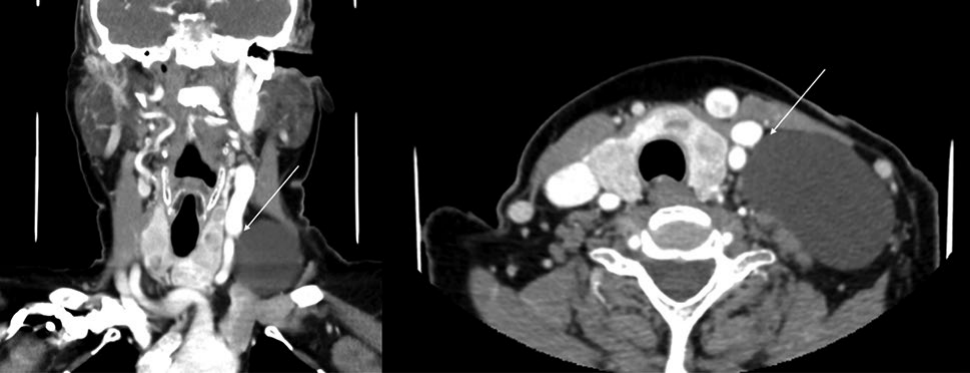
Coronal and axial contrast CT images, demonstrating a homogenous cystic lesion in the left supraclavicular region, in close relationship to the head and neck vasculature
